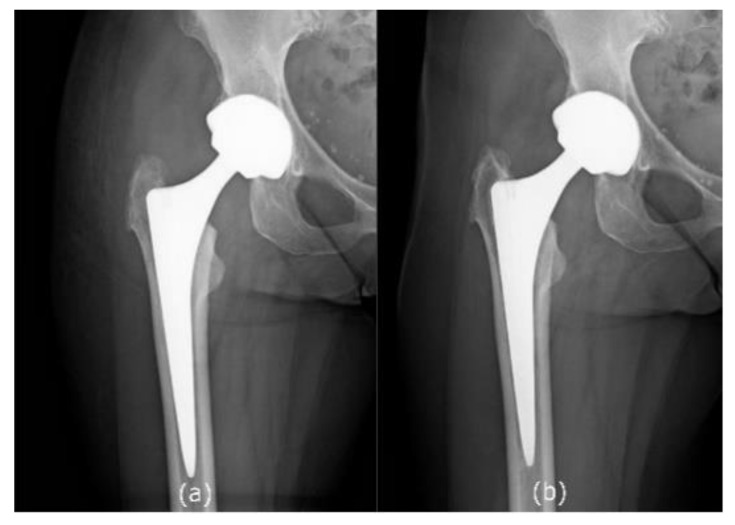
V.D., female, 58 - year-old, Ceramic-on-Ceramic Total Hip arthroplasty for femoral neck fracture, right hip X-ray: (a) Post-operative follow-up, one month (b) Post-operative follow-up, 5 years (24 months after recruitment).
